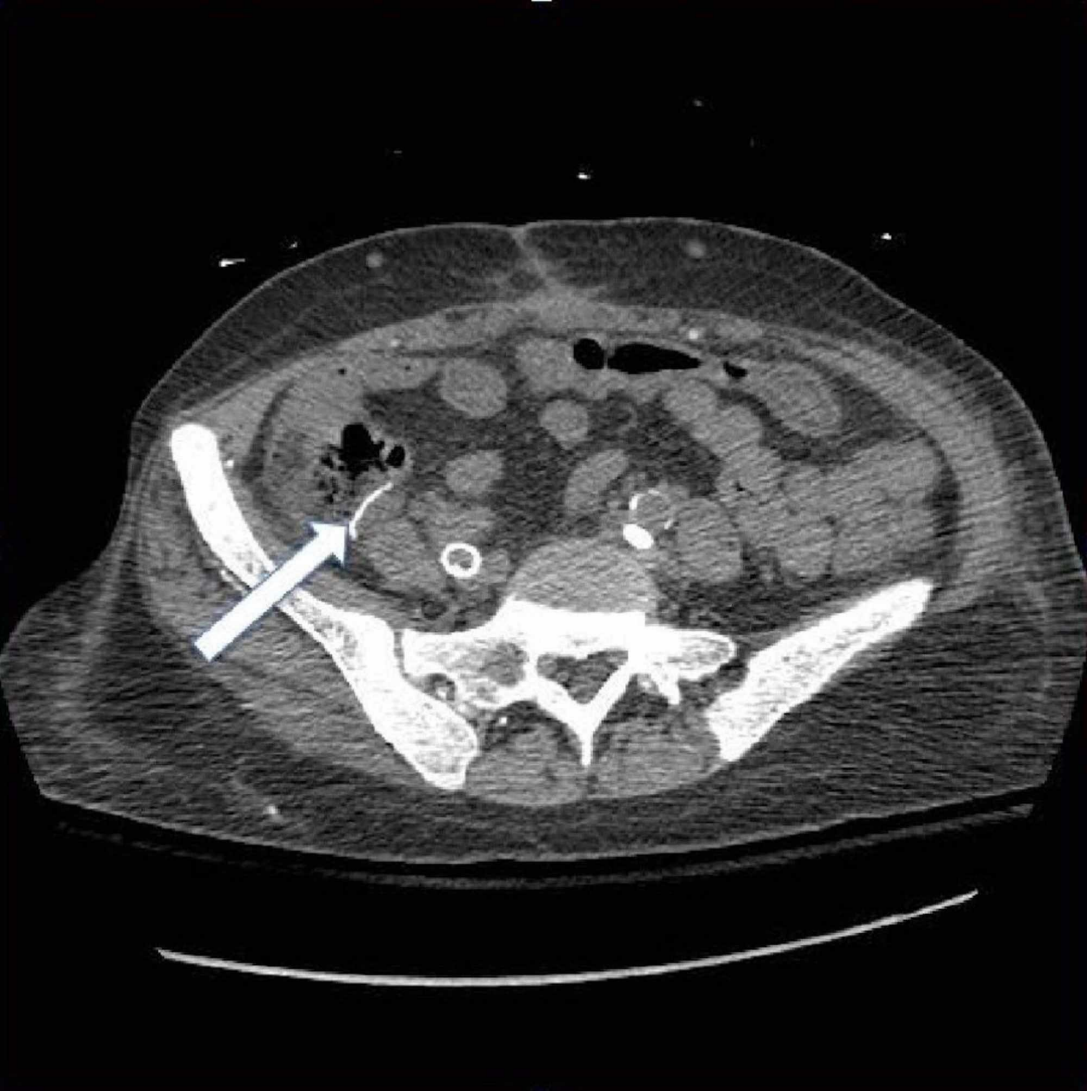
CT of the abdomen and pelvis without contrast findings showing anastomosis in the right lower quadrant, which appears to be intact with no definitive enterocolitis.
